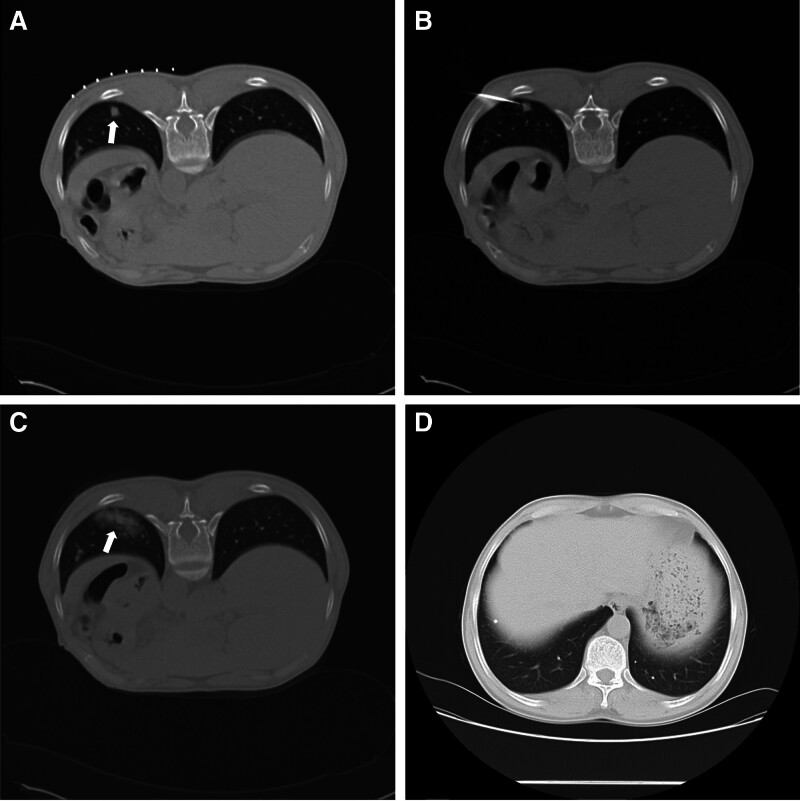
(A) A 57-yr-old male patient exhibited an 11 mm inflammatory nodule in the left lower lobe (white arrow). (B) CT-guided PCNB was performed using an 18-gauge cutting needle. (C) Transpulmonary needle pathway with alveolar hemorrhage after PCNB (white arrow). (D) 6 mo later, the pulmonary nodules had disappeared. CT = computed tomography, PCNB = percutaneous core needle biopsy.
